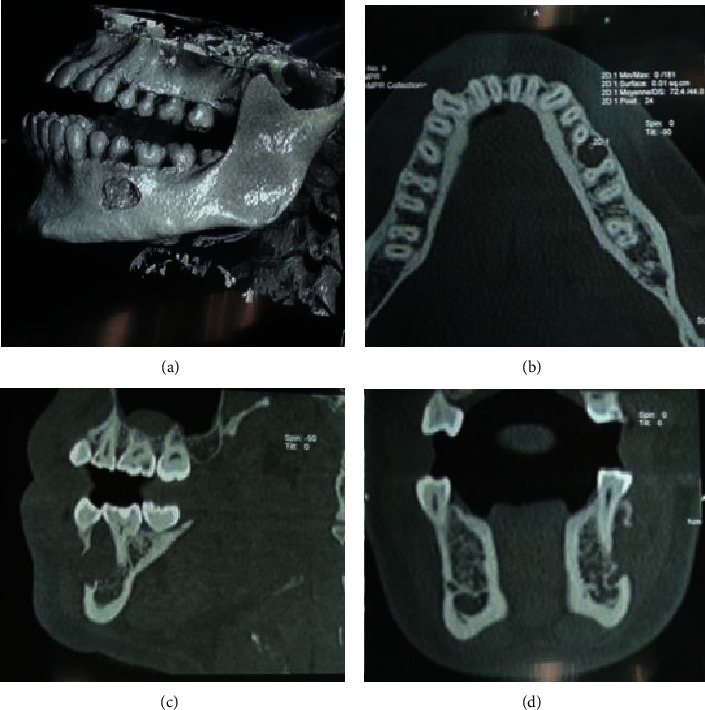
Dentascan radiography presenting the lesion on the left mandible on (a): a 3D reconstruction; (b): an axial view; (c): a sagittal view; and (d): a coronal view.
